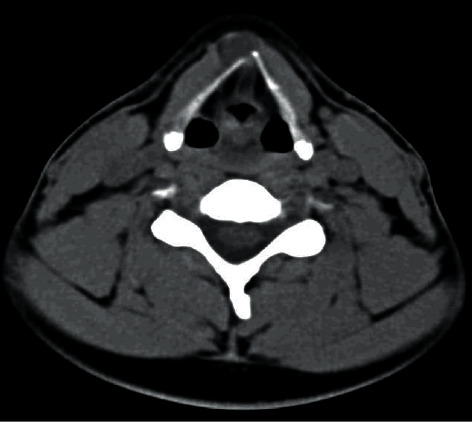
Axial cross section of a soft-tissue window CT image of the TGDC.
